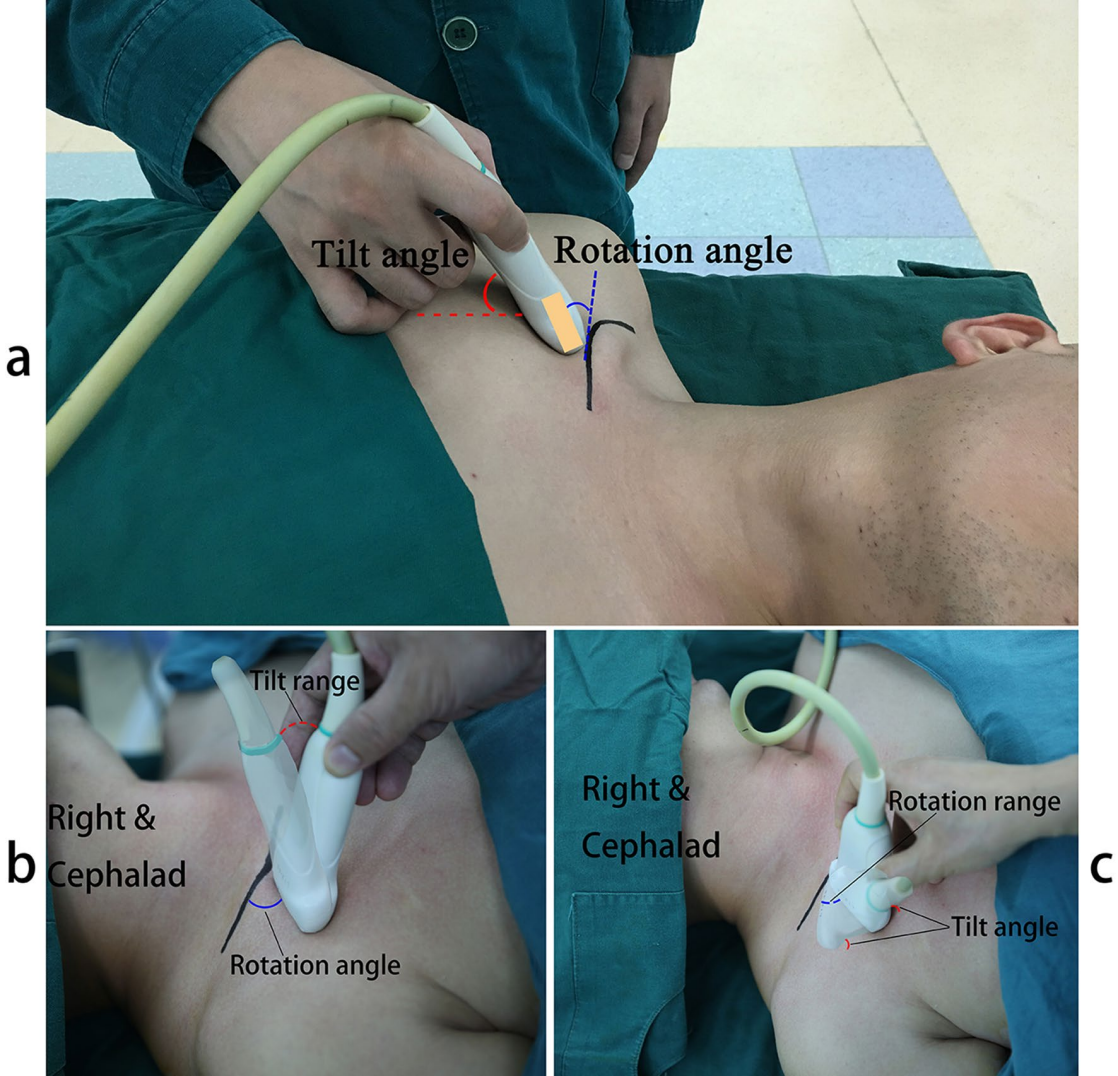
The rotation and tilt angle of ultrasound probe and the acceptable range of probe manipulation. (a) The rotation (green) angle between long axis of probe and clavicle; and the tilt (red) angle between ultrasonic beam plane and right chest-wall plane. (b) The acceptable range of probe tilt before the clear visualization of SCV disappeared. The optimal rotation angle was fixed. (c) The acceptable range of probe rotation before the clear visualization of SCV disappeared. The optimal tilt angle was fixed.
